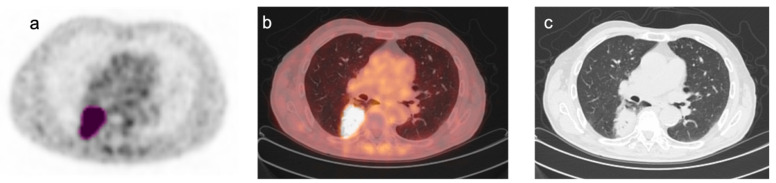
Representative images of [^18^F]FDG PET/CT scan in a patient with NSCLC. Representative images of [^18^F]FDG PET/CT scan in a patient with NSCLC: (a) transaxial [^18^F]FDG PET image; (b) co-registered PET/CT fusion image; (c) co-registered CT image. A tridimensional region of interest was drawn around the FDG avid tumor lesion (pink) using an automated contouring program setting an absolute threshold for SUV at 2.5 (a). Fusion and CT images confirmed the accuracy of lesion segmentation (b,c).
